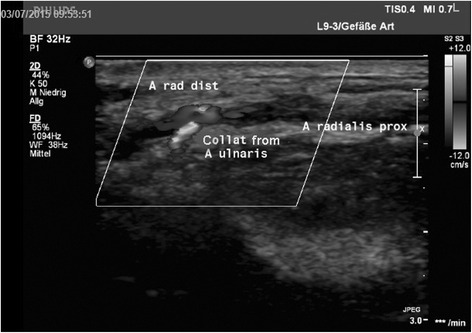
Color coded Doppler sonography shows origin of the ‚palpabel pulse’ in the distal radial artery (A rad dist): Due to a collateral from the ulnar artery (Collat from A ulnaris) a pulse was palpabel in the distal radial artery even though the proximal radial artery (A radialis prox) was occluded
